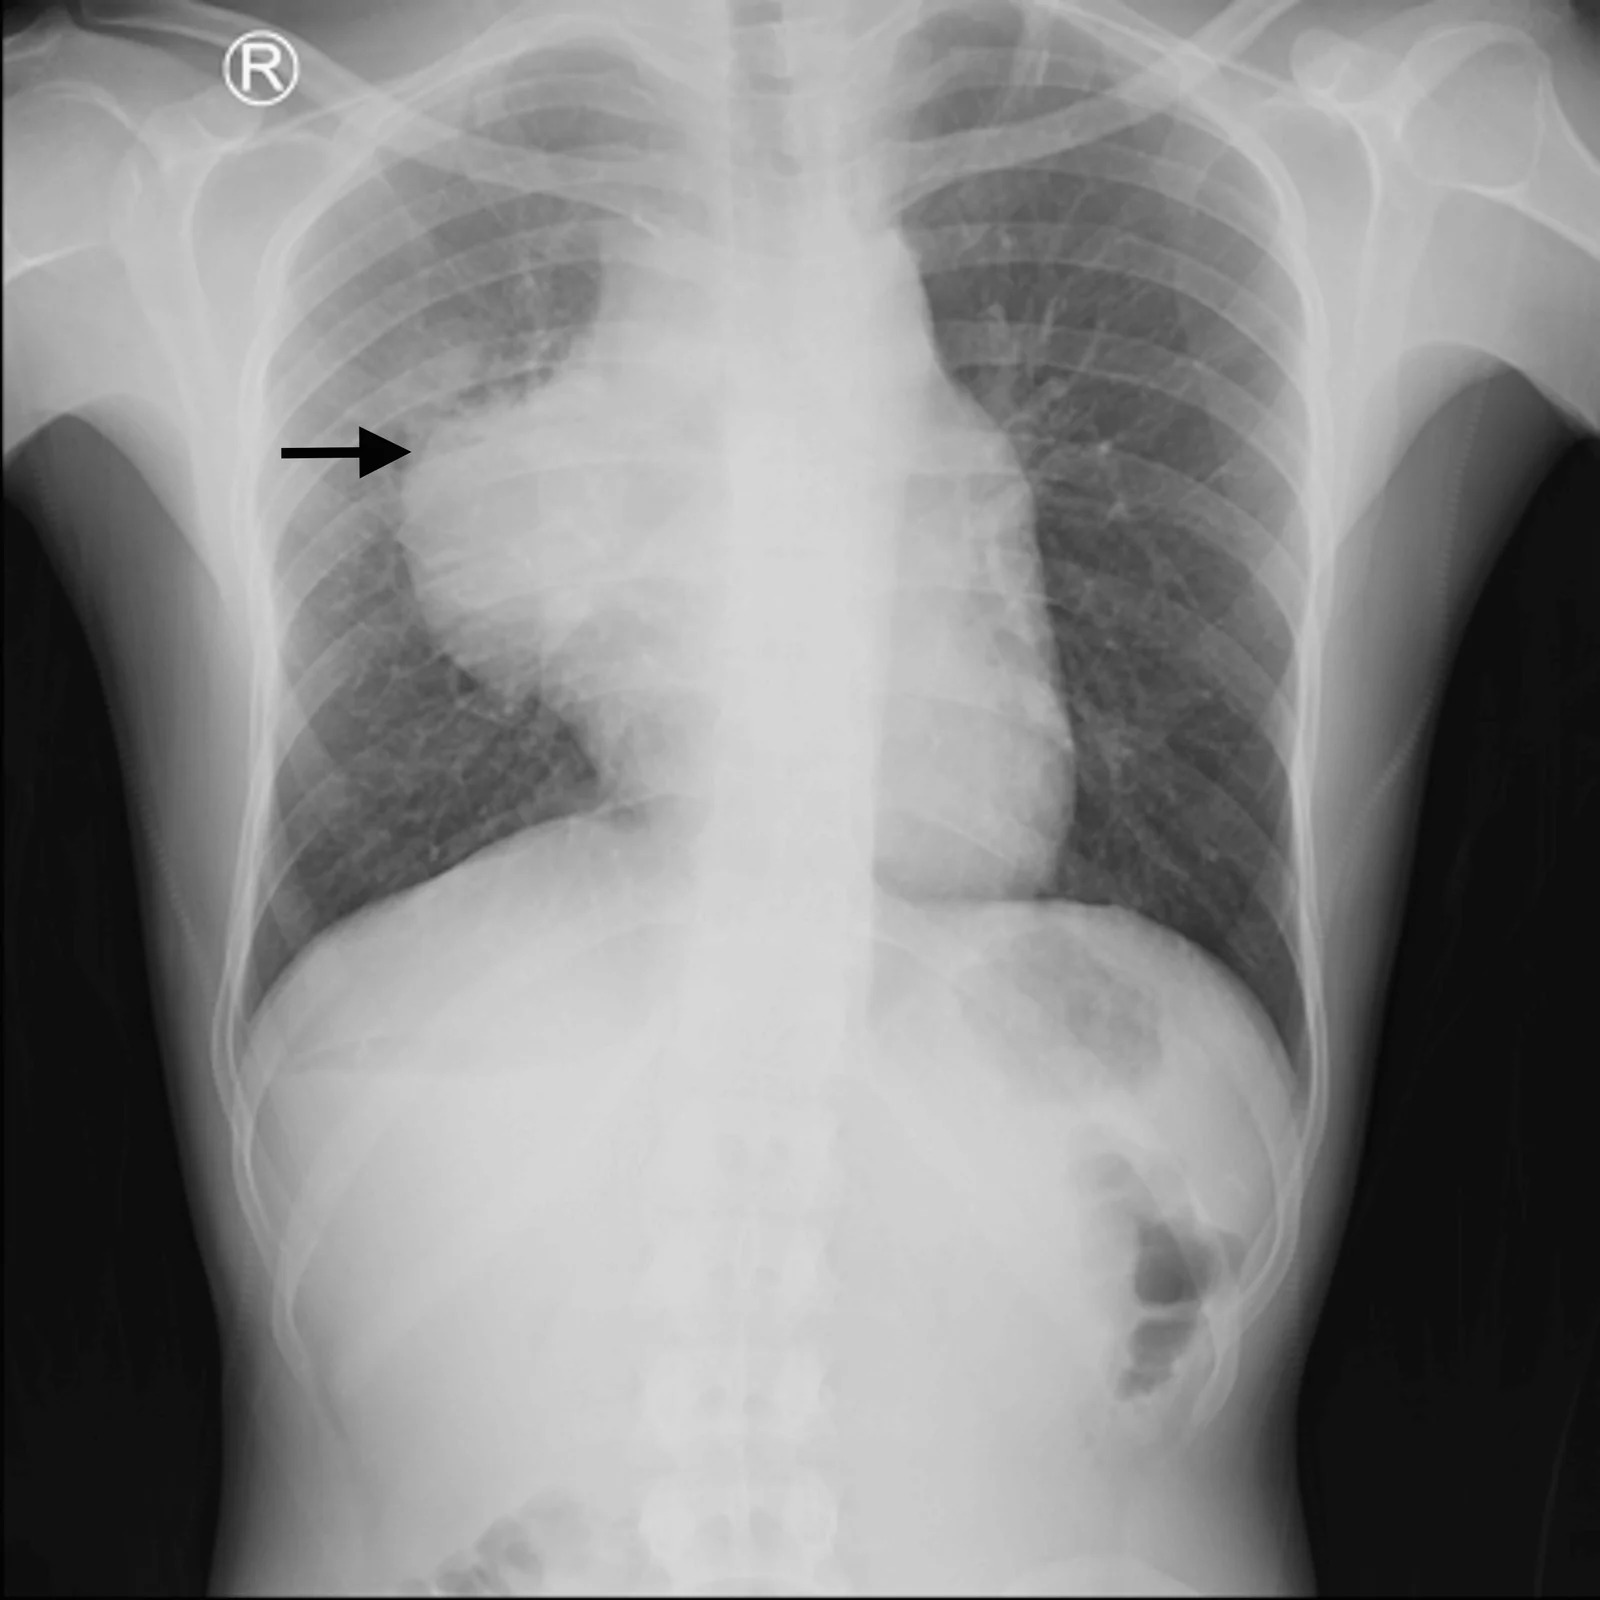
Chest X-ray showing right-sided mediastinal opacity mass (arrow)
